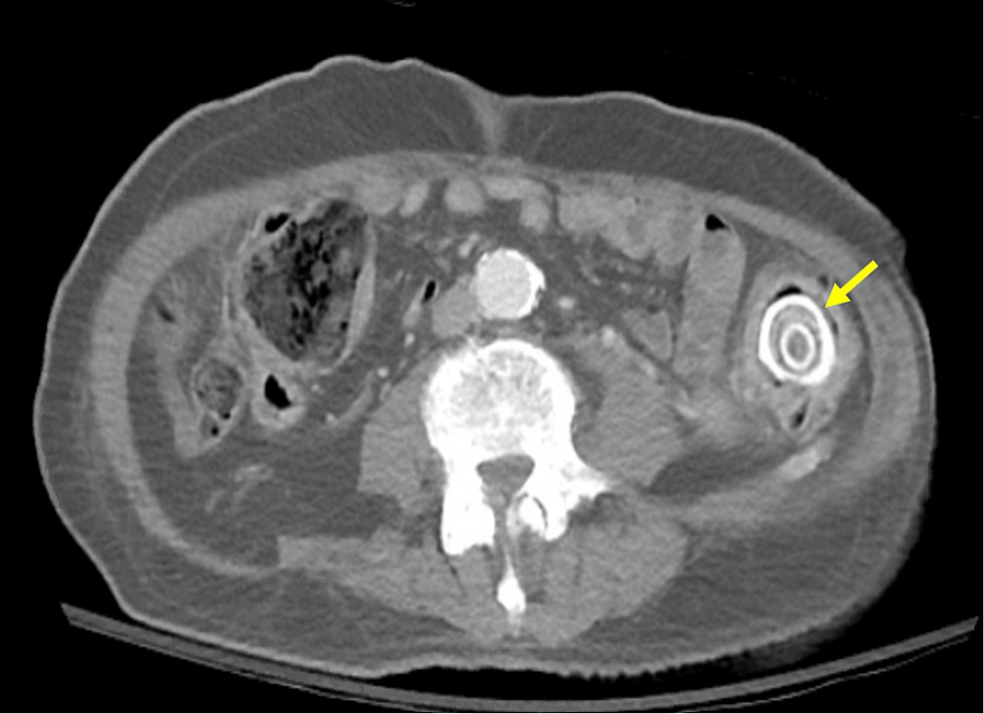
CT abdomen pelvis shows large lamellated stone in the distal descending colon.
